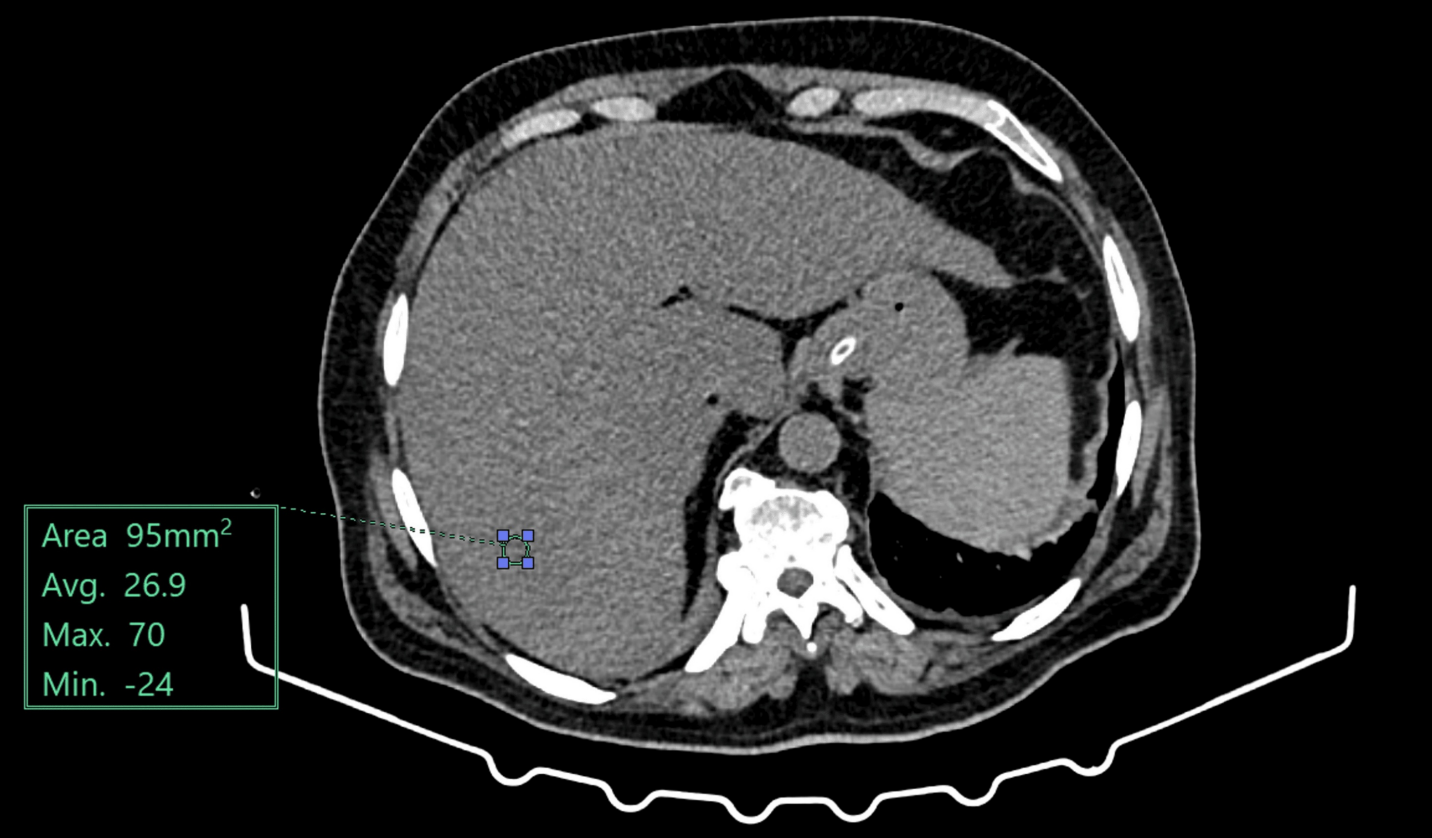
CT image of fatty liver with Hounsfield unit value
